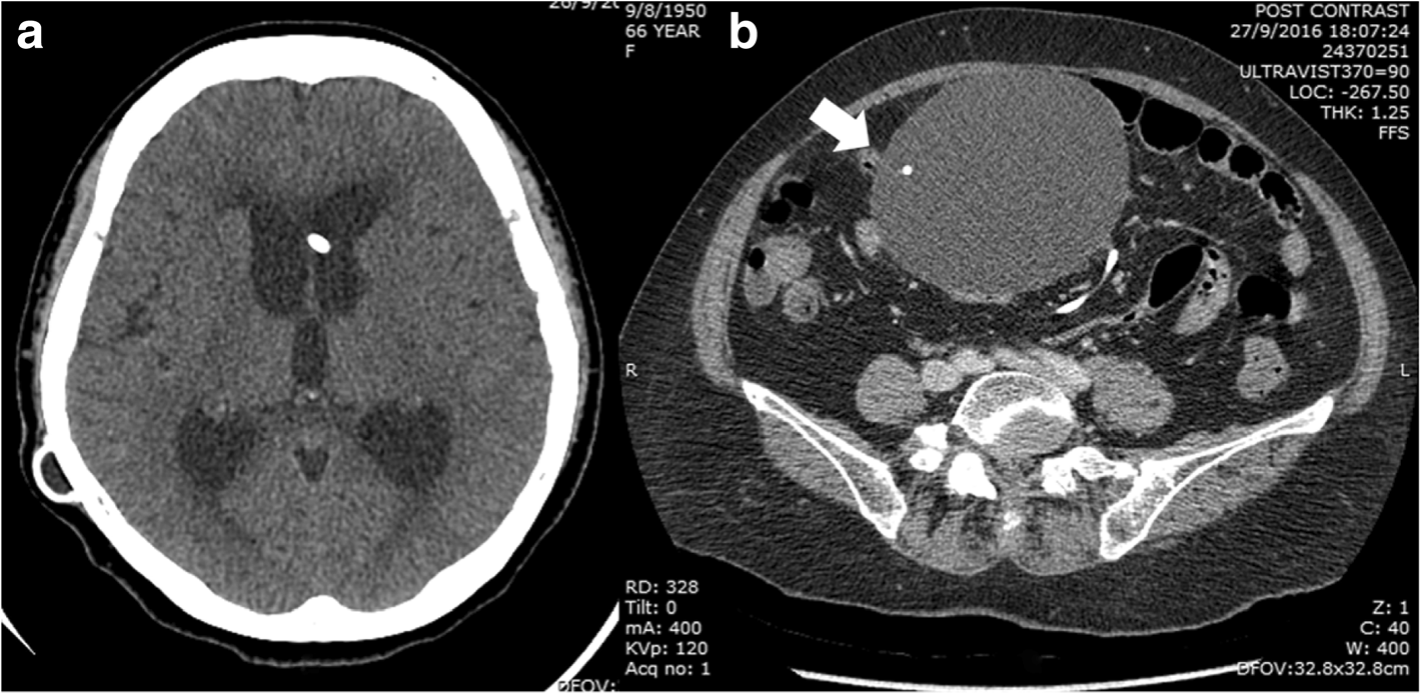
a) Computed tomography of brain revealed recurrent communicating hydrocephalus with appropriate in-place VP shunt, b) Computed tomography of whole abdomen with contrast revealed a large well-defined cystic mass at inframesocolic space, approximately 9.6 × 11.3 × 15 cm in diameter which it encased the distal limb of VP shunt (arrow)
